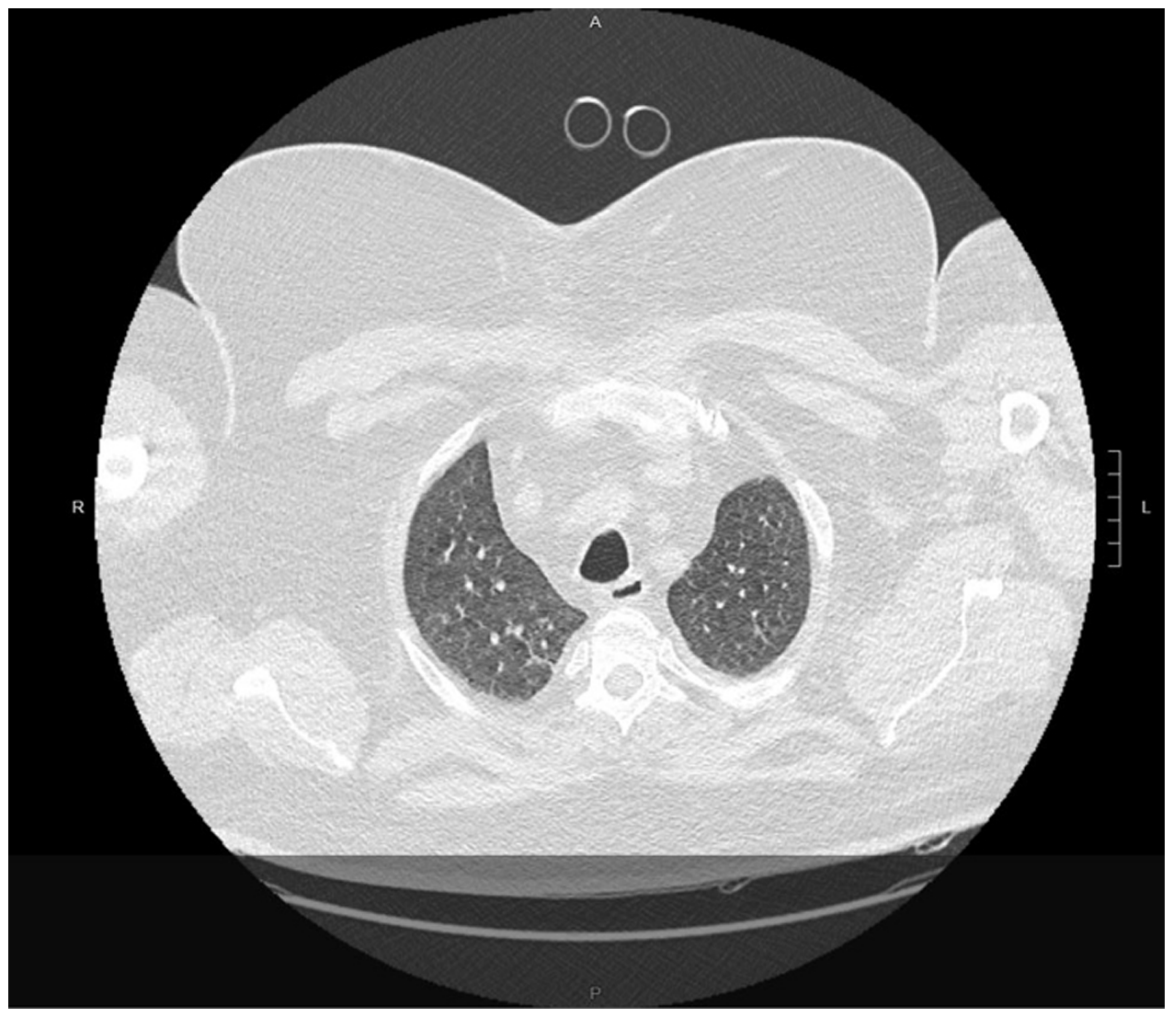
Follow-up chest computed tomography after clinical improvement. Marked resolution of the parenchymal consolidations and ground-glass opacities is observed following supportive and antimicrobial therapy, consistent with recovery from hMPV-related pneumonia.
